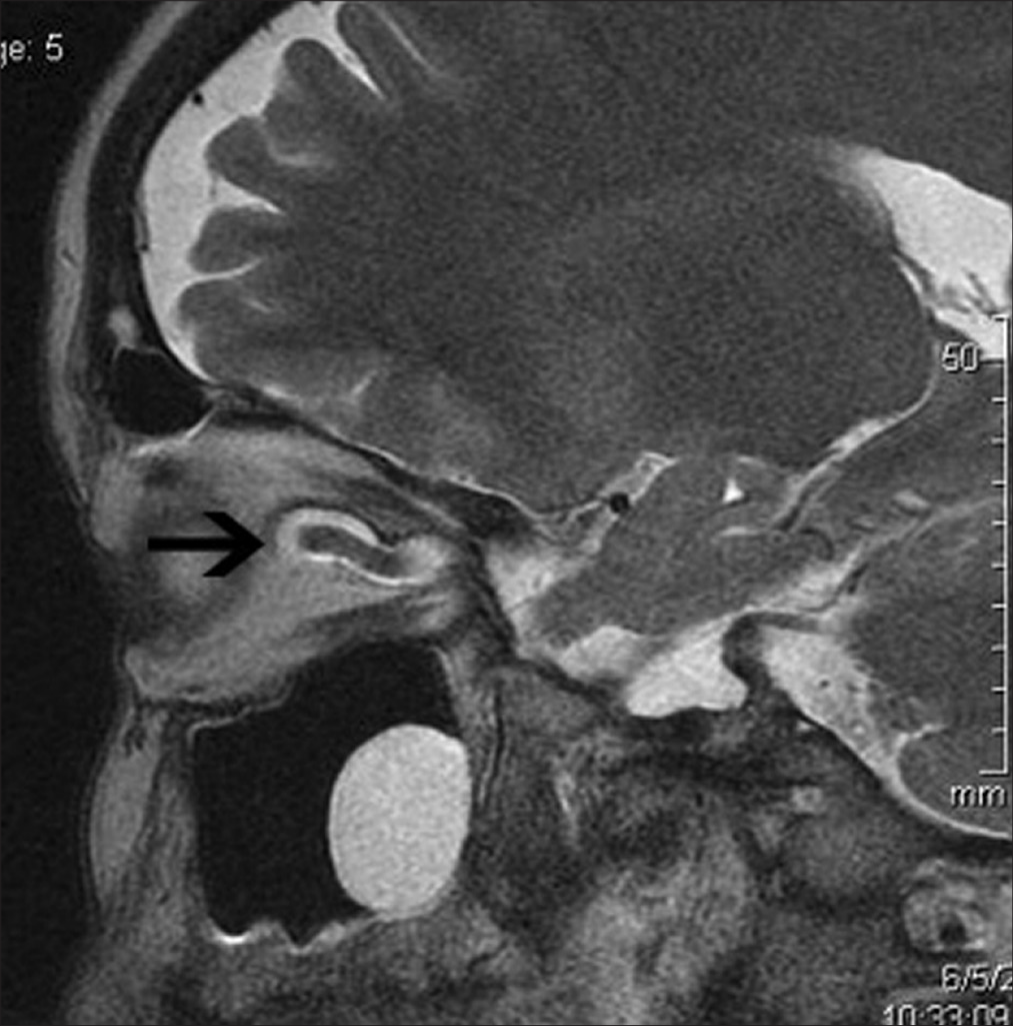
Sagittal T2-weighted image shows vertical tortuosity of optic nerve ( black arrow)
